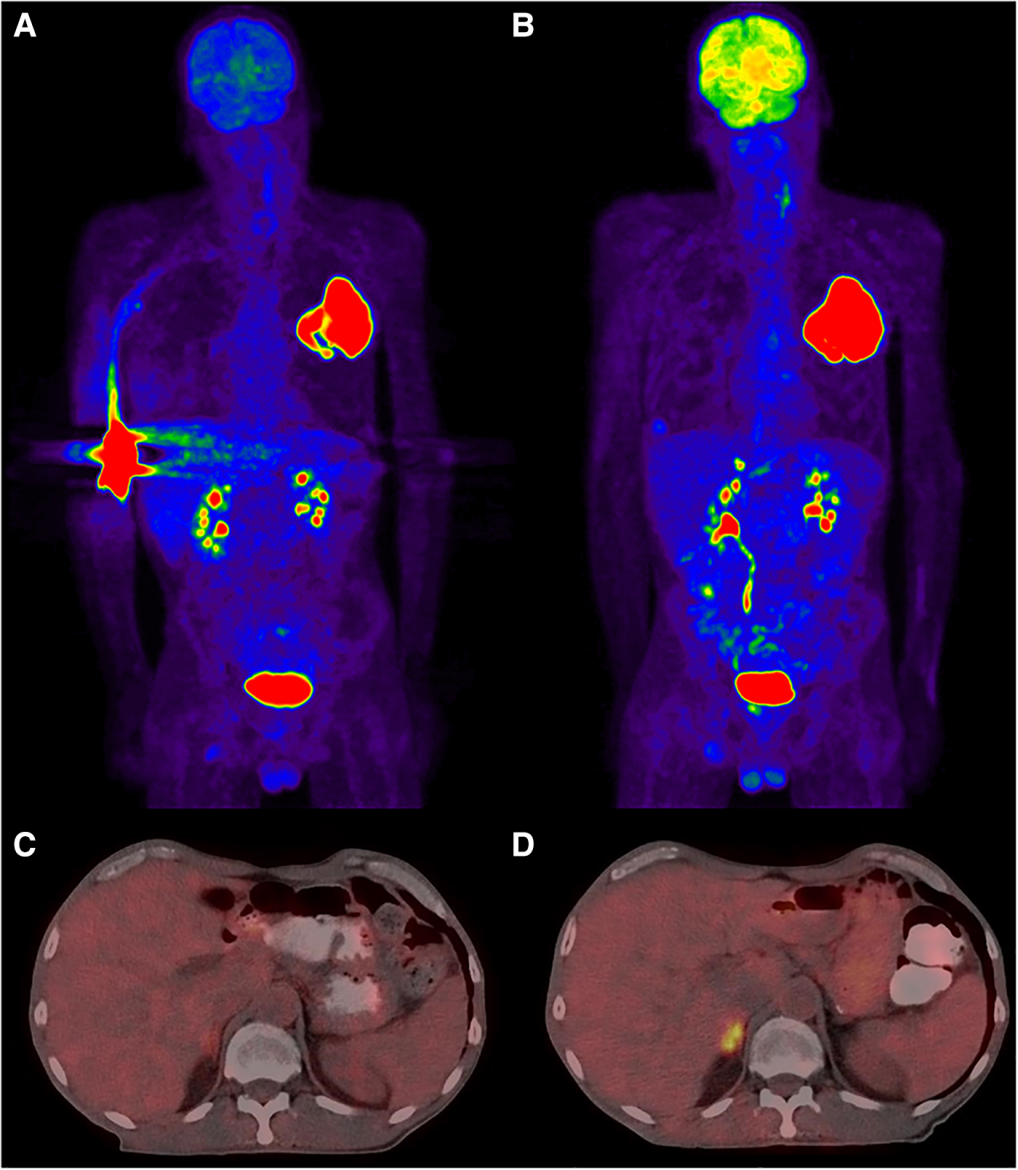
PET/CT images demonstrating the implications of a large extravasation. Subparts (A) and (C) correspond to the extravasated imaging, (B) and (D) are from reimaging three days later with no extravasation. Subpart (A): maximum intensity projection (MIP) showing extravasation in the right antecubital and a large mass in the upper left lung. Subpart (C): slice at the renal level indicating no obvious occult uptake. Subpart (B): MIP view now with an additional lesion evident in the adrenals. Subpart (D): slice from the renal level showing increased focal uptake within the adrenals.
